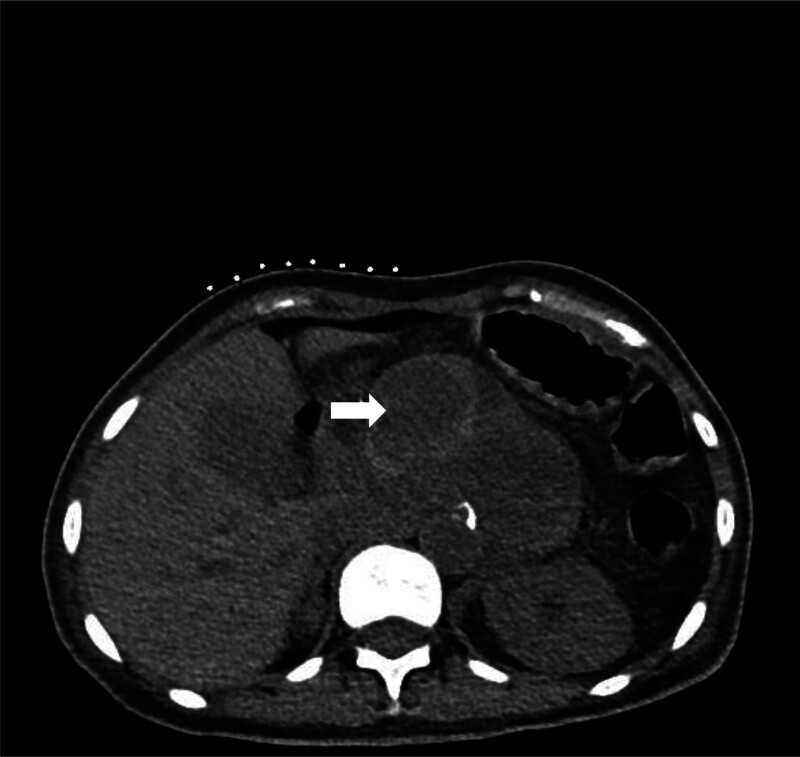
Unenhanced CT scan before the procedure showing a round, slightly high-density lesion (arrow).
